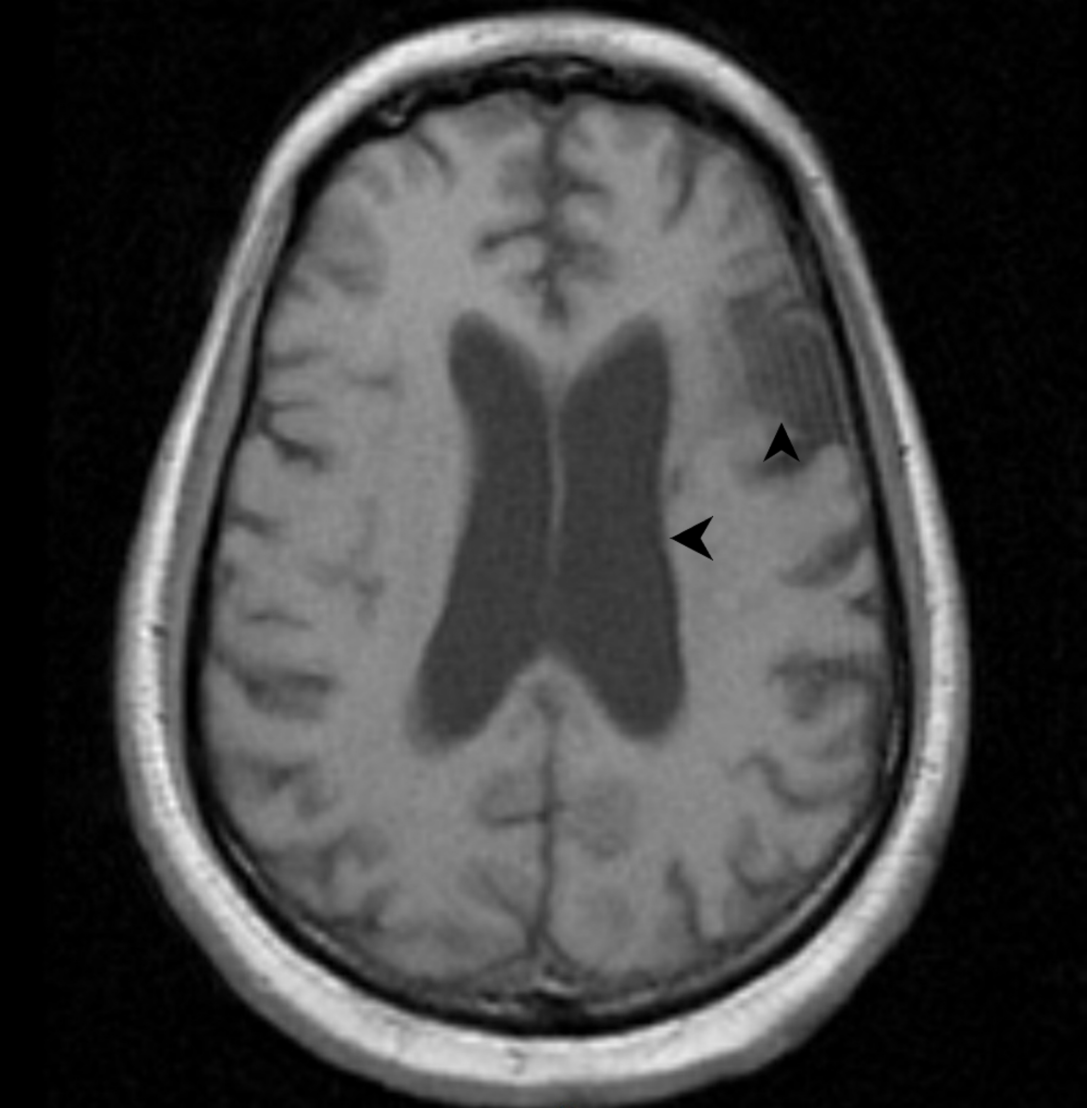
T1-weighted MRI scan of the brain (without contrast) also showing an enlarged left lateral ventricle and encephalomalacia in the left middle cerebral artery territory (black arrows).
